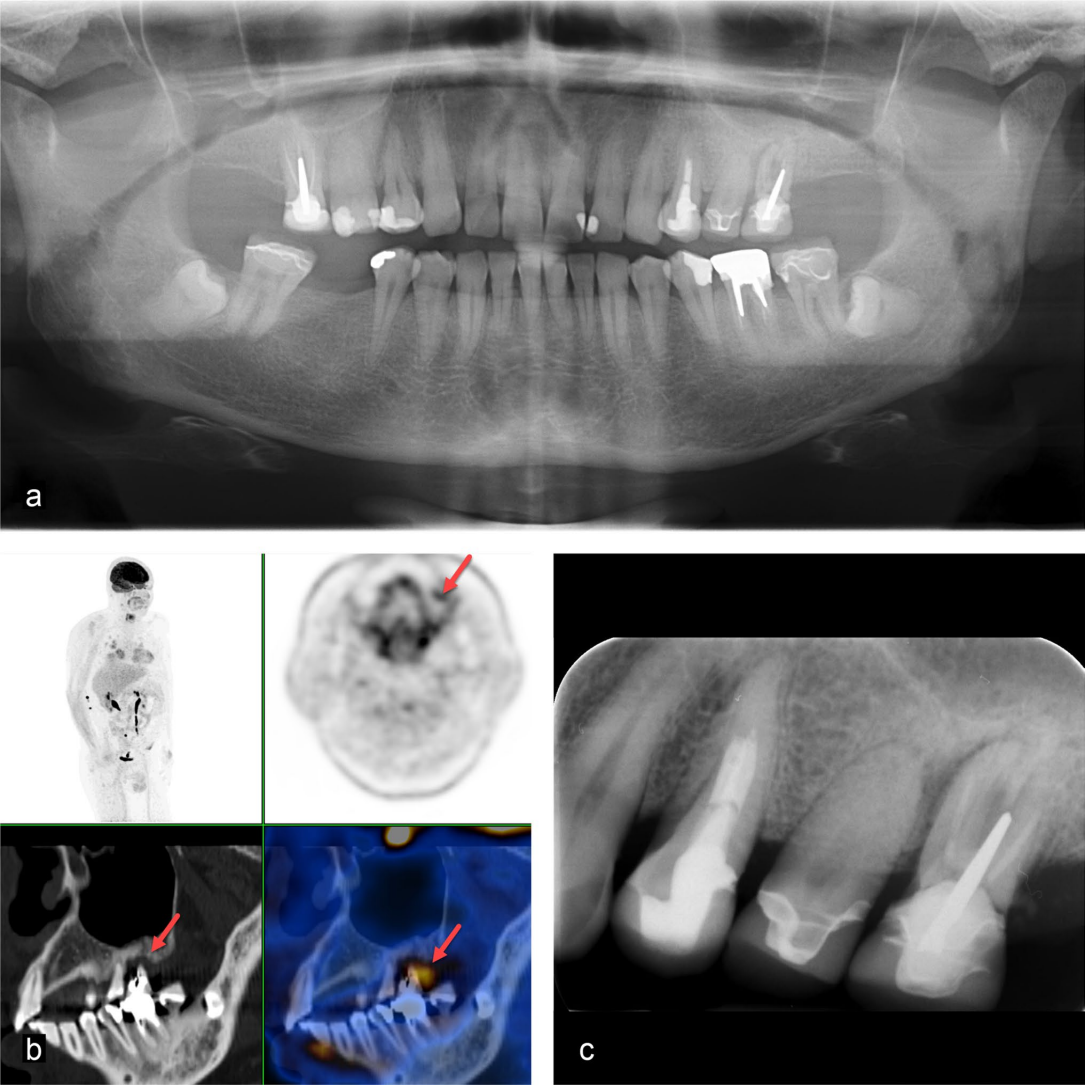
A 60-year-old woman with left-sided hypopharynx carcinoma cT2 cN0 cMx. Clinical examination showed increased mobility, probing depth, and a positive percussion test of tooth 26: a Panoramic radiography visualises impacted third molars in the mandible. b FDG-PET/CT shows a metabolically active osteolysis around tooth 26 with a SUV_max_ of 6.6 (red arrow). c The periapical radiograph visualises a combined perio-endo problem of tooth 26. The respective tooth was extracted, dental hygiene performed, and a fluoride splint prepared
